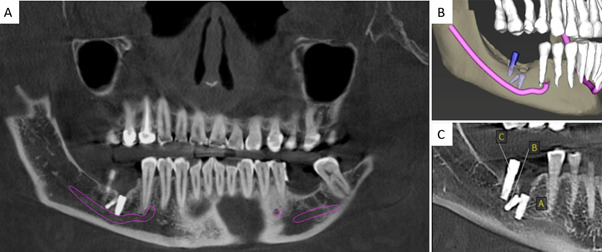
A) Reconstrucción panorámica de tomografía computarizada cone beam. B) Reconstrucción 3D de CBCT con un trazado del nervio dentario inferior. C) Corte sagital de CBCT que evidencia la relación de los implantes dentales (A, B y C) con el nervio dentario inferior.
